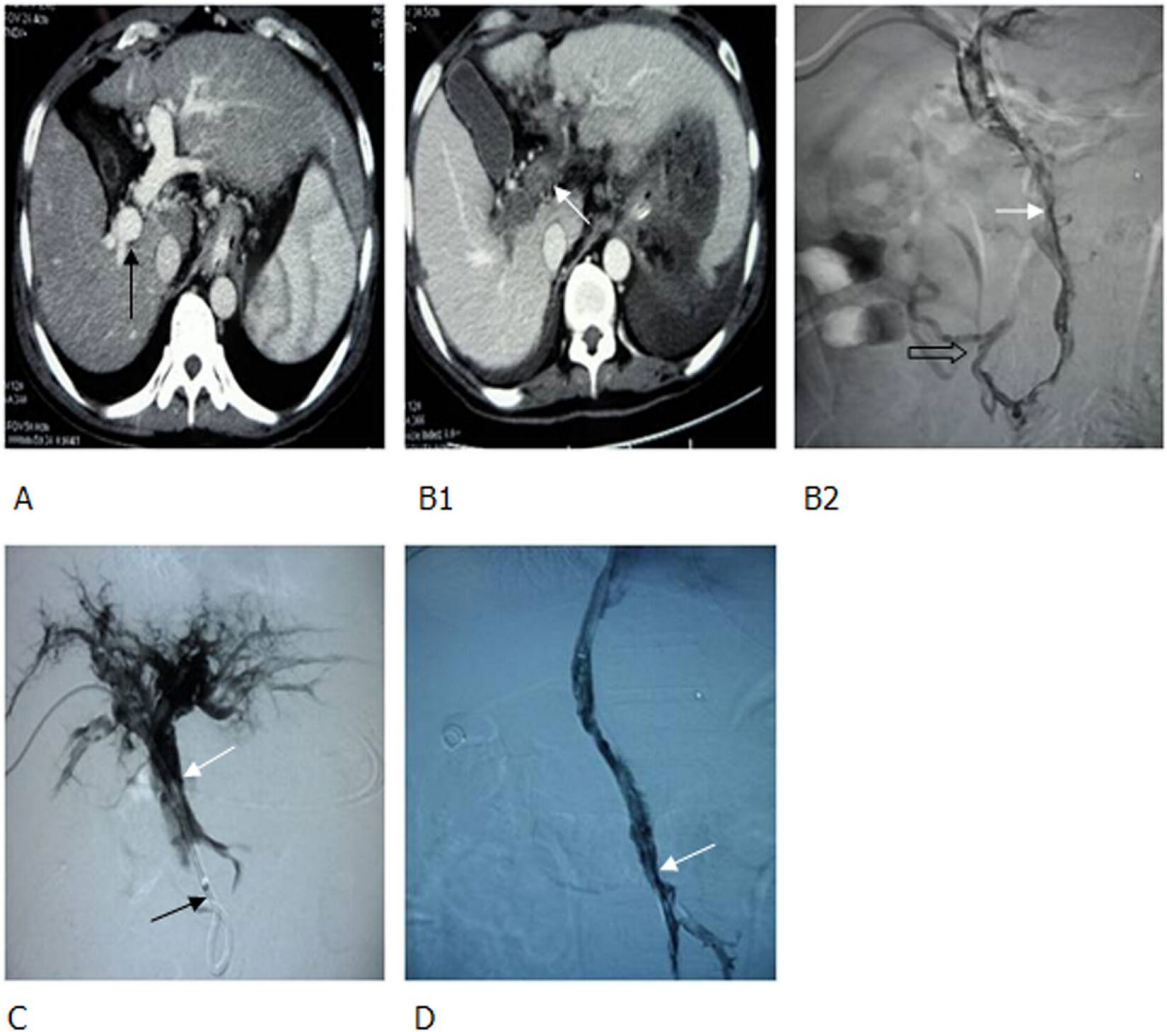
Cirrhotic portal hypertension and total thrombosis in portal vein 5 days after splenectomy, percutaneous transhepatic embolectomy and thrombectomy; 2 days after local thrombolysis and anticoagulant therapy, complicated with gastrointestinal bleeding. (A) Before splenectomy, enhanced CT scan demonstrated that portal vein was completely unblocked (black arrow), without any thrombus. (B1, B2) At the third and fifth day following splenectomy, enhanced CT scan and percutaneous transhepatic portography identified total thrombi in portal vein and superior mesenteric vein (white arrow). A few collateral vessels of superior mesenteric vein were present (hollow arrow). (C) After embolectomy, thrombectomy was conducted with a balloon, local thrombolysis, and anticoagulant therapy. Thus, thrombi in portal vein were decreased (white arrow), however, thrombi in superior mesenteric vein were not significantly changed (black arrow), complicated with gastrointestinal bleeding. (D) After establishing a shunt using 2 covered stents during TIPS, portography demonstrated the shunt, as well as unblocked MPV and superior mesenteric vein. However, some thrombi remained in portal vein (white arrow). CT, computed tomography.
